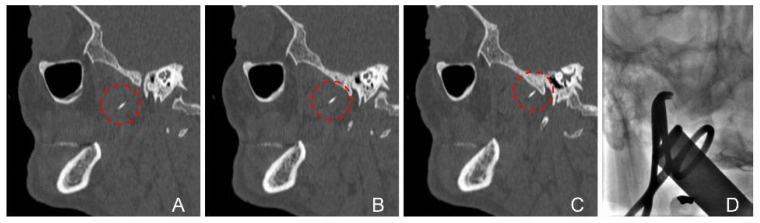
CT sagittal images showing syringe needle in the masticatory space (A–C) and surgical removal with intraoperative C-arm X-ray (D). The foreign body is indicated by a dotted circle.
